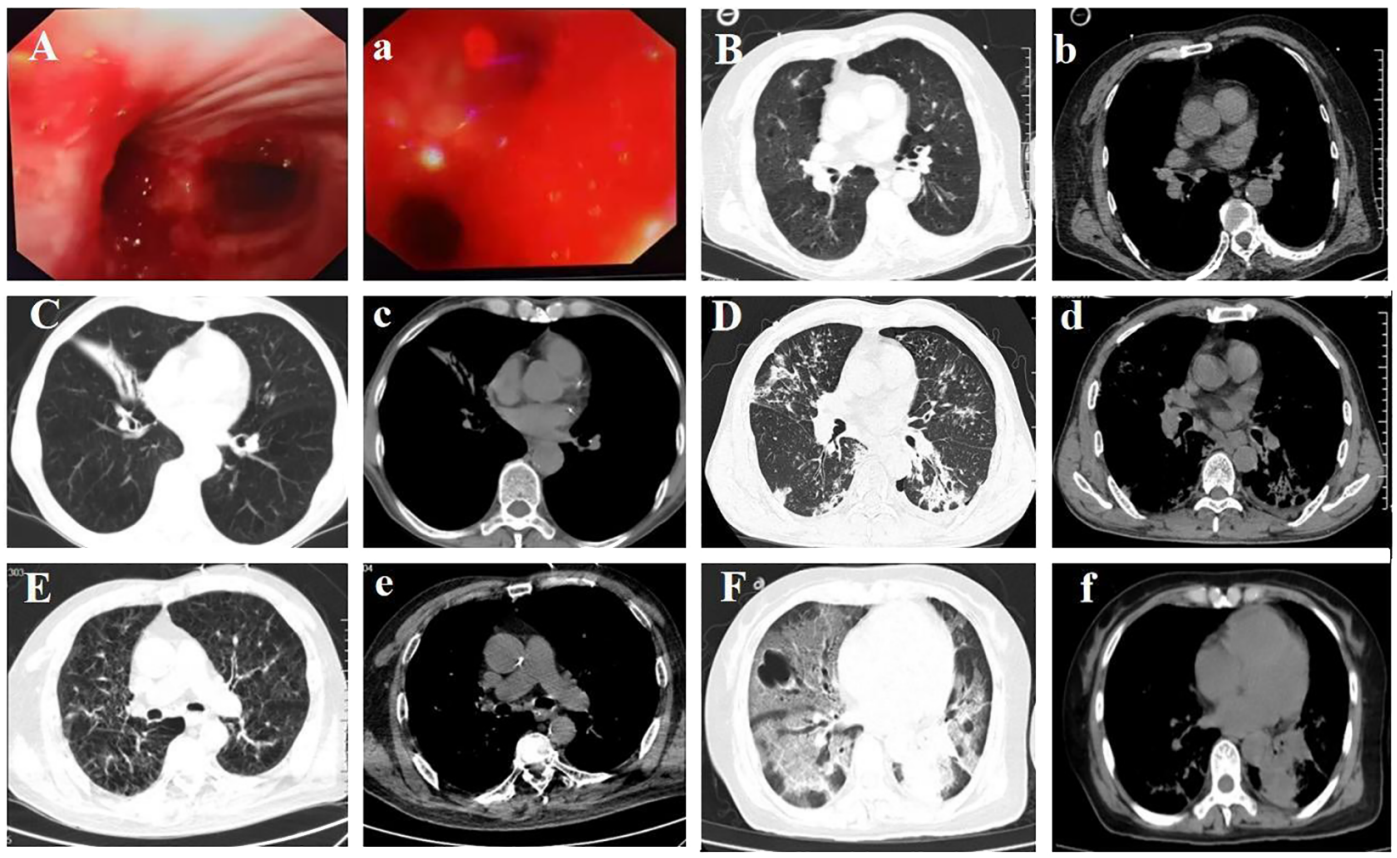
Diffuse alveolar hemorrhage and several typical lung CT findings of IAV pneumonia. (Aa). Diffuse alveolar hemorrhage caused by IAV infection, as observed through fiberoptic bronchoscopy. (Bb). IAV infection co-occurring with *Mycobacterium hominis* and *Streptococcus pneumoniae* infections. (Cc). Patients with COPD and IAV infection complicated by *Pseudomonas aeruginosa* infection. (Dd). Patients with COPD and IAV infection complicated by *Candida albicans* infection. (Ee, Ff). IAV infection co-occurring with *Aspergillus* infection. CT, computed tomography; IAV, influenza A virus; COPD, chronic obstructive pulmonary disease, SP, *Streptococcus pneumoniae.*.
